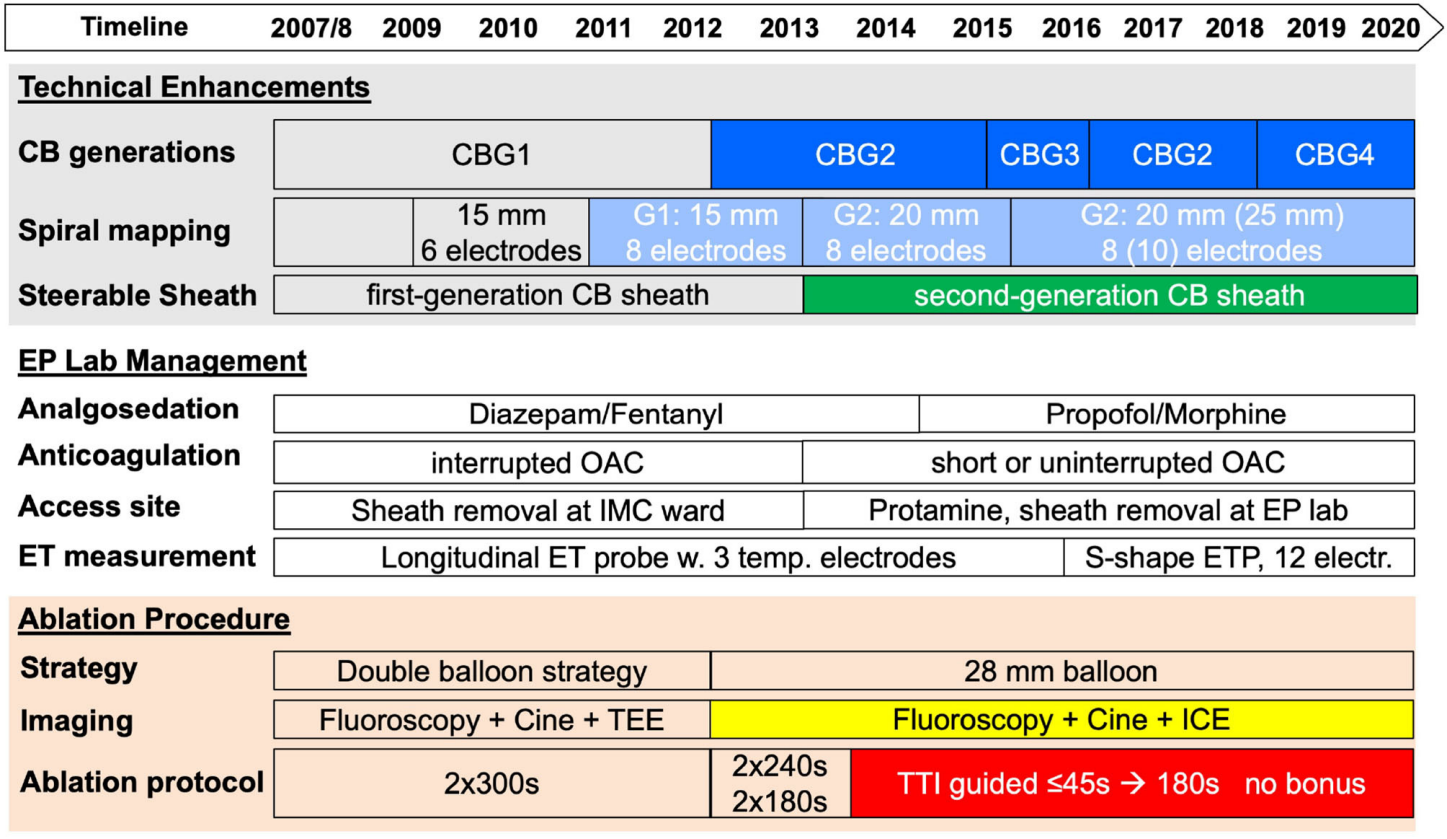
Evolution of cryoballoon ablation for persistent atrial fibrillation (AF) from 2007 to 2020. A timeline of technical enhancements, improvement in laboratory management, and implementation of new cryoballoon protocols are shown in the upper part of the figure. CB, cryoballoon; CBG, cryoballoon generation; EP, electrophysiology; ET, esophageal temperature; ICE, intracardiac echocardiography; TEE, transesophageal echocardiography; TTI, time-to-isolation.
